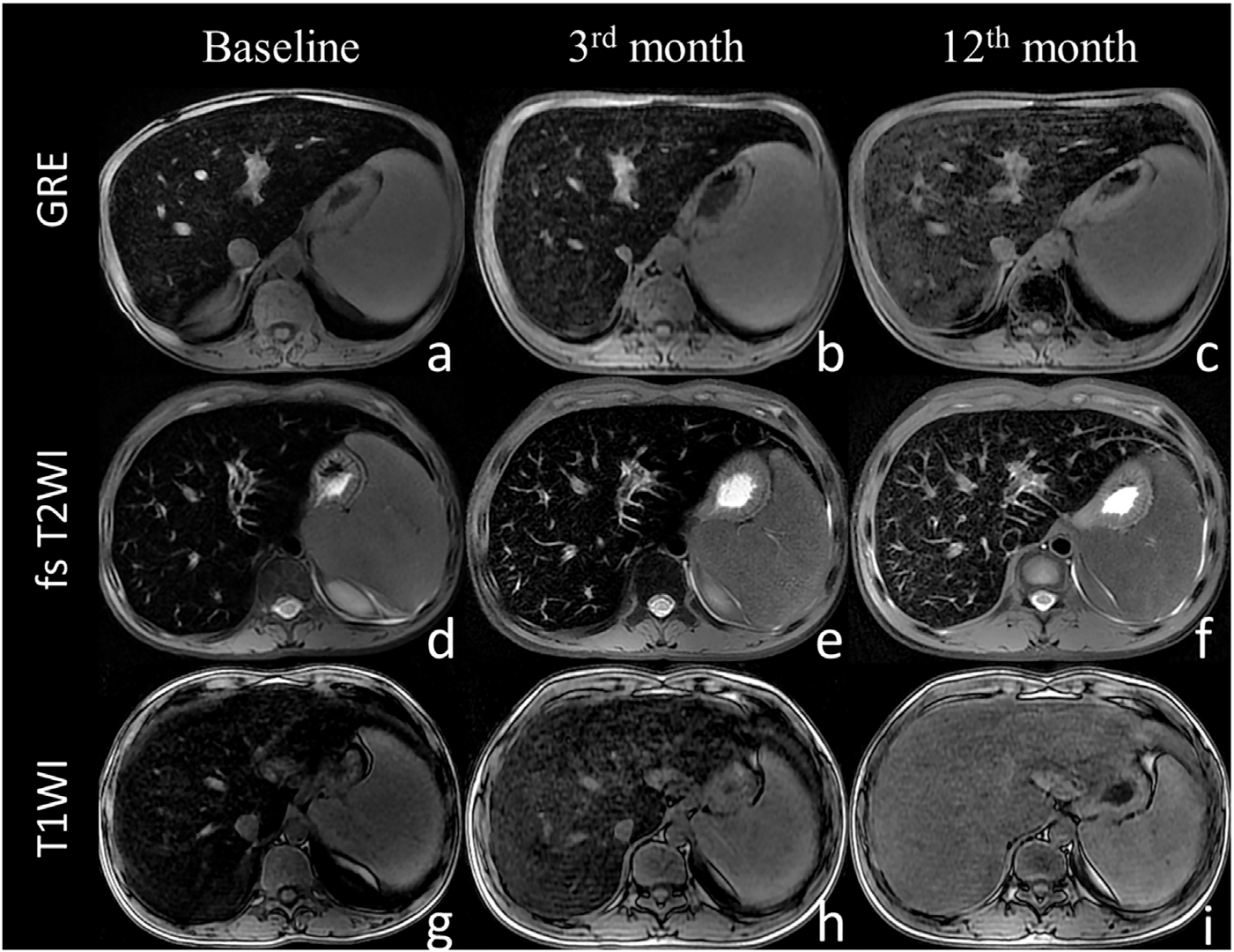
The liver magnetic resonance imaging signal intensity with the sequence of gradient echo (A–C), fast spin echo sequence T2-weighted imaging (D–F) and T1-weighted imaging (G–I) at baseline (A,D,G), 3 months (B,E,H), and 12 months (C,F,I). The liver MRI signal intensity increased with thalidomide treatment during the 12 months of follow up.
